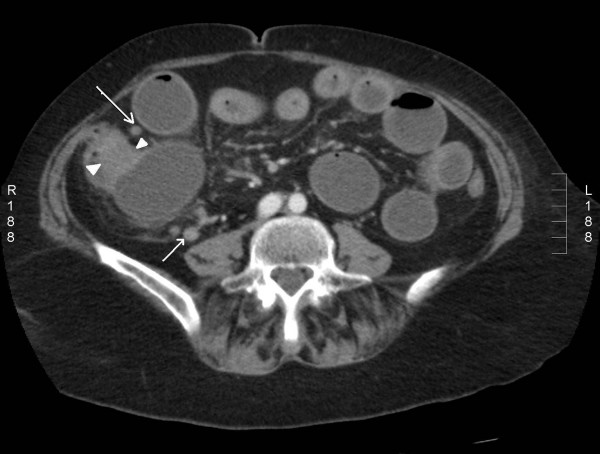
An axial CT image showing dilated small bowel loops and concentric thickening of caecal wall (arrow heads) close to the ileocaecal junction. Pericolic and ileocolic lymphnodes are also seen (arrow).
